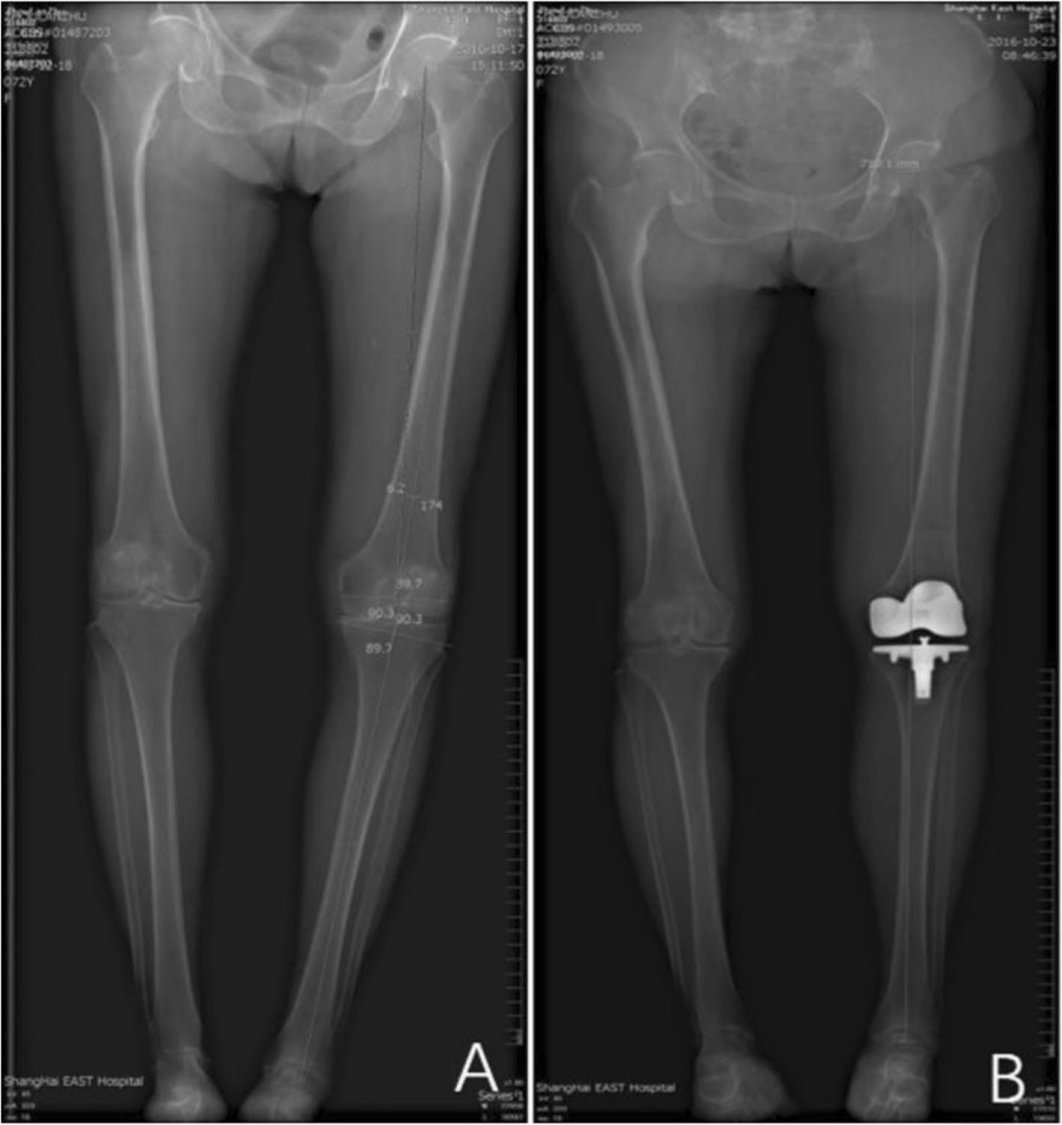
Pre- and post-operative long leg standing anteroposterior X-ray images. a Pre-operative X-ray shows the distal lateral femur angle, the proximal medial tibial angle, and the mechanical axis. b Postoperative X-ray shows a neutral mechanical axis
